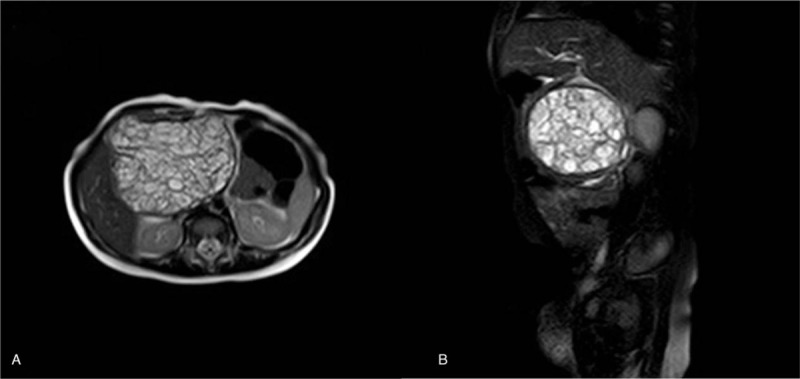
(A, B). Preoperative MRI scan of the pediatric pancreatic teratoma. Tumors contain a large amount of fat and sebum, and the outer membrane is intact. MRI = magnetic resonance imaging.
